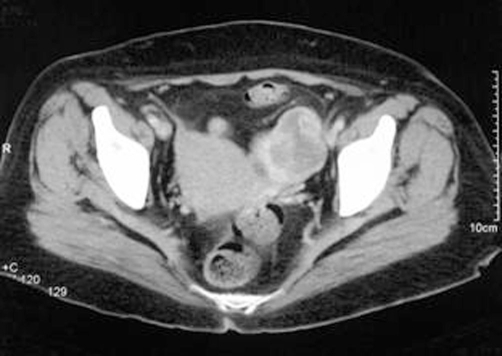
Contrast CT scan of the Left Ovarian metastasis; showing a 4 cm heterogeneous mass with fairly intense enhancement.
